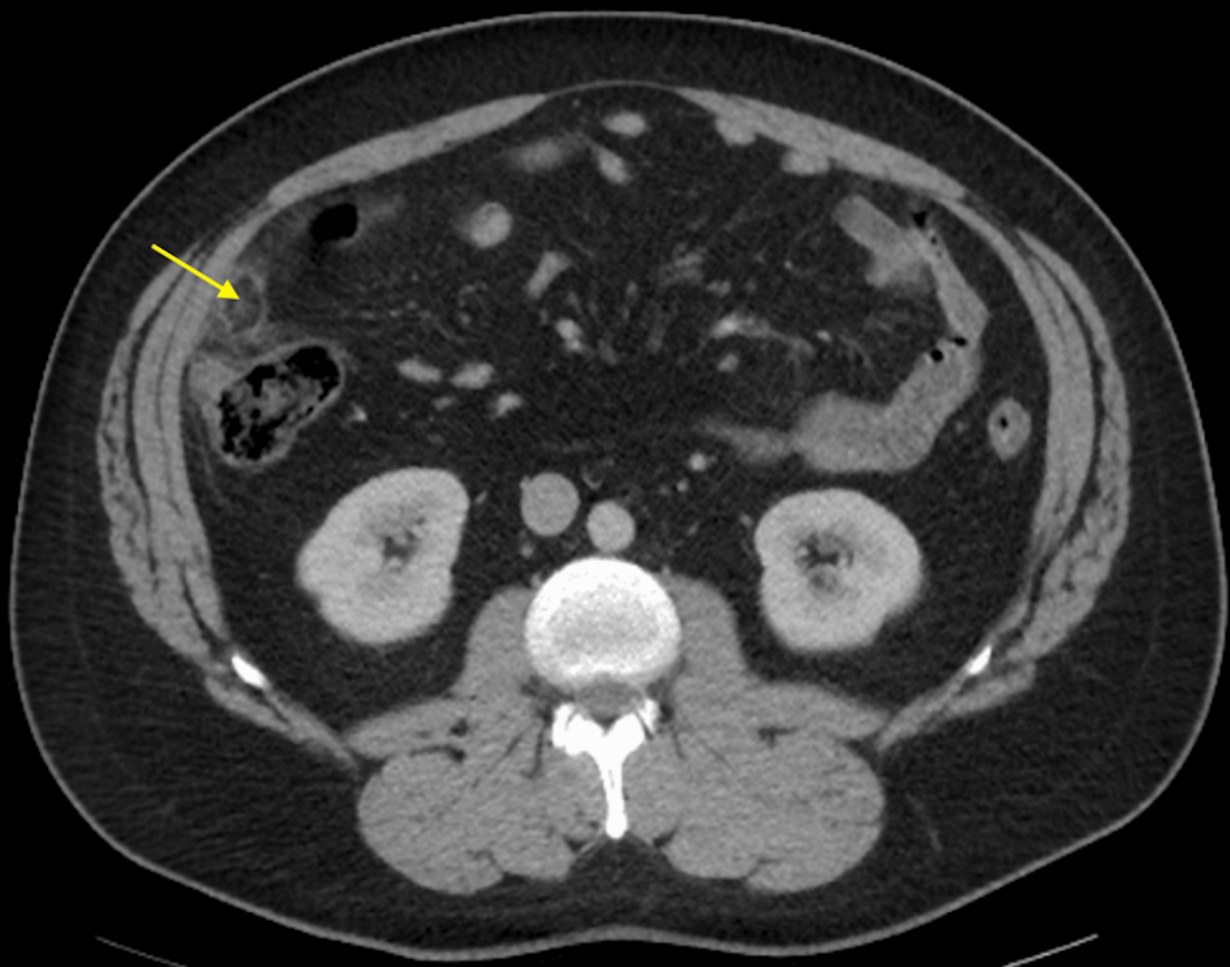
Computed Tomography (CT) scan with highlighted epiploic appendagitis of the ascending colon
